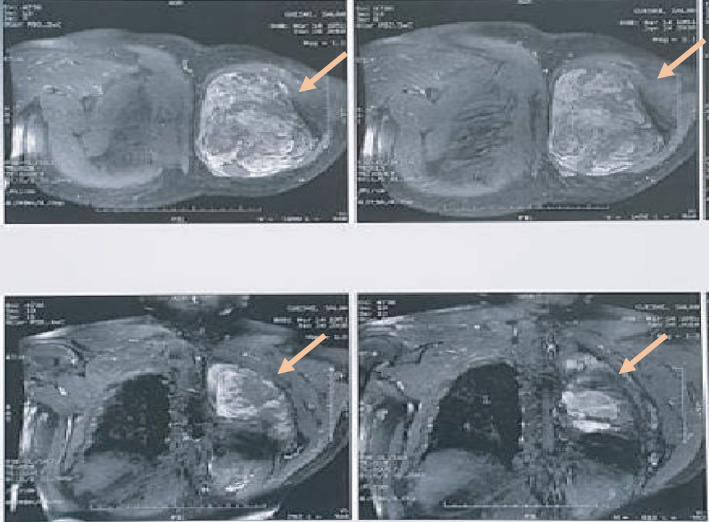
MRI T1 saturated postcontrast (gadolinium) sequence of the dorsal soft tissues showing a malignant tumor of 18.5*20 cm within the left para‐vertebral muscles and invading the posterior arch of the 5th and 6th ribs
